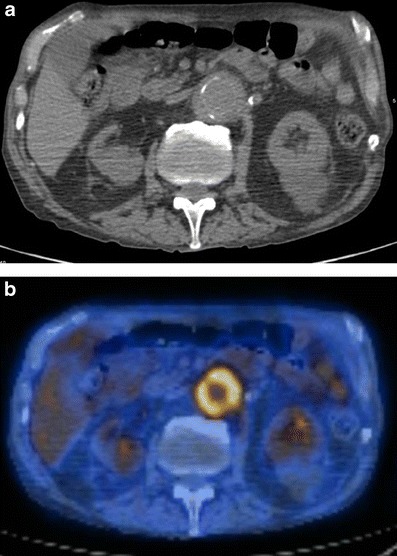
A 75-year-old male with abdominal pain. An axial non-contrast-enhanced CT image (a) of the abdomen demonstrates circumferential homogeneous thickening of the aortic wall up to 5 mm corresponding to vivid circumferential 18-FDG uptake on PET-CT image (b). Subsequent biopsy of the aortic wall was consistent with idiopathic inflammation
